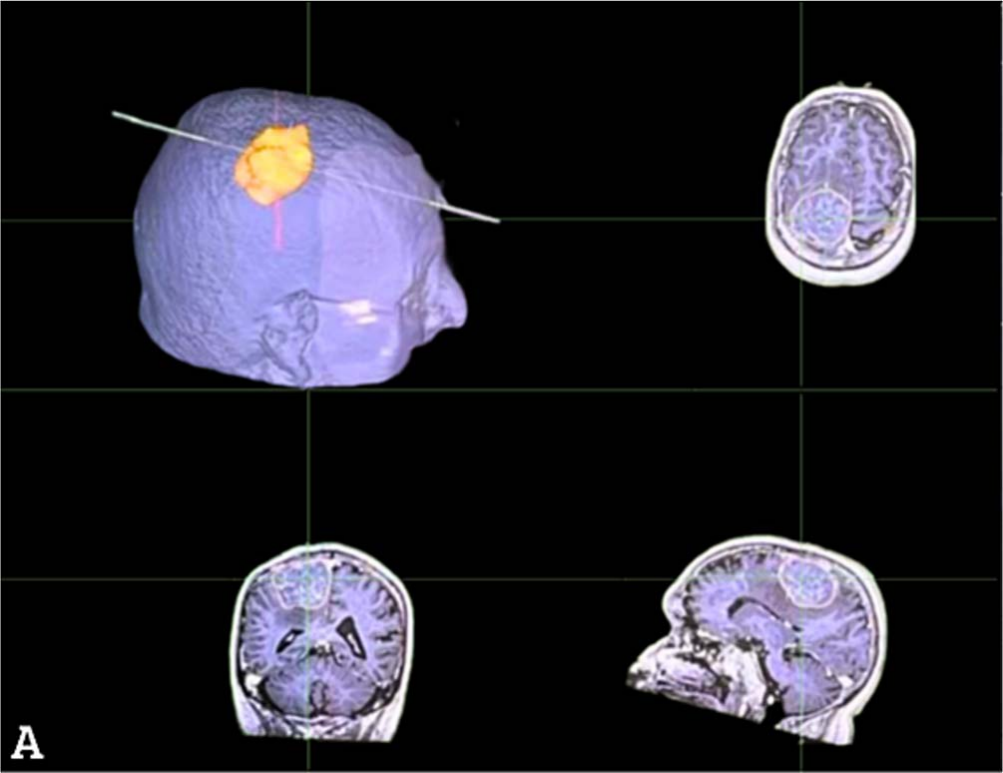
Perioperative neuronavigation. Display MRI sequences with axial, coronal and sagittal views and a 3D reconstruction of the tumor in relation to the skull aiding in surgical planning and demonstrating the tumor’s size.
